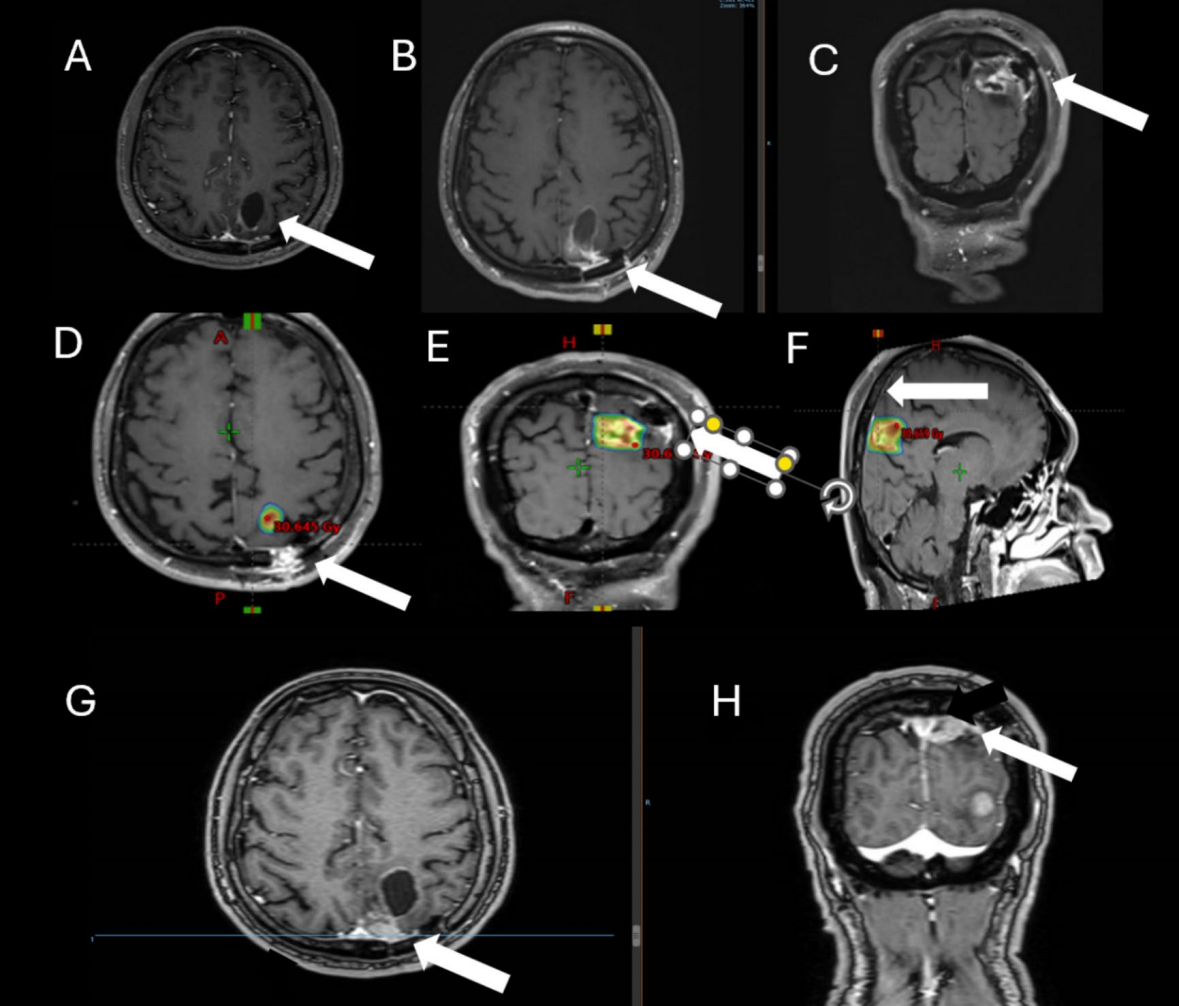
Brain MRI of a 59-year-old diagnosed with triple negative breast cancer. Presenting symptoms of brain metastasis was episode. Brain MRI showing a left parietooccipital cystic lesion (A). She underwent craniotomy (B, C – MRI of the surgical cavity) and the resection cavity was treated to a total dose of 25 Gy in 5 fractions not including the corridor and all surgical cavity (D, E, F, arrow). At 10 months she presented with local failure near the corridor (arrow) and a new brain metastasis (G, H)
